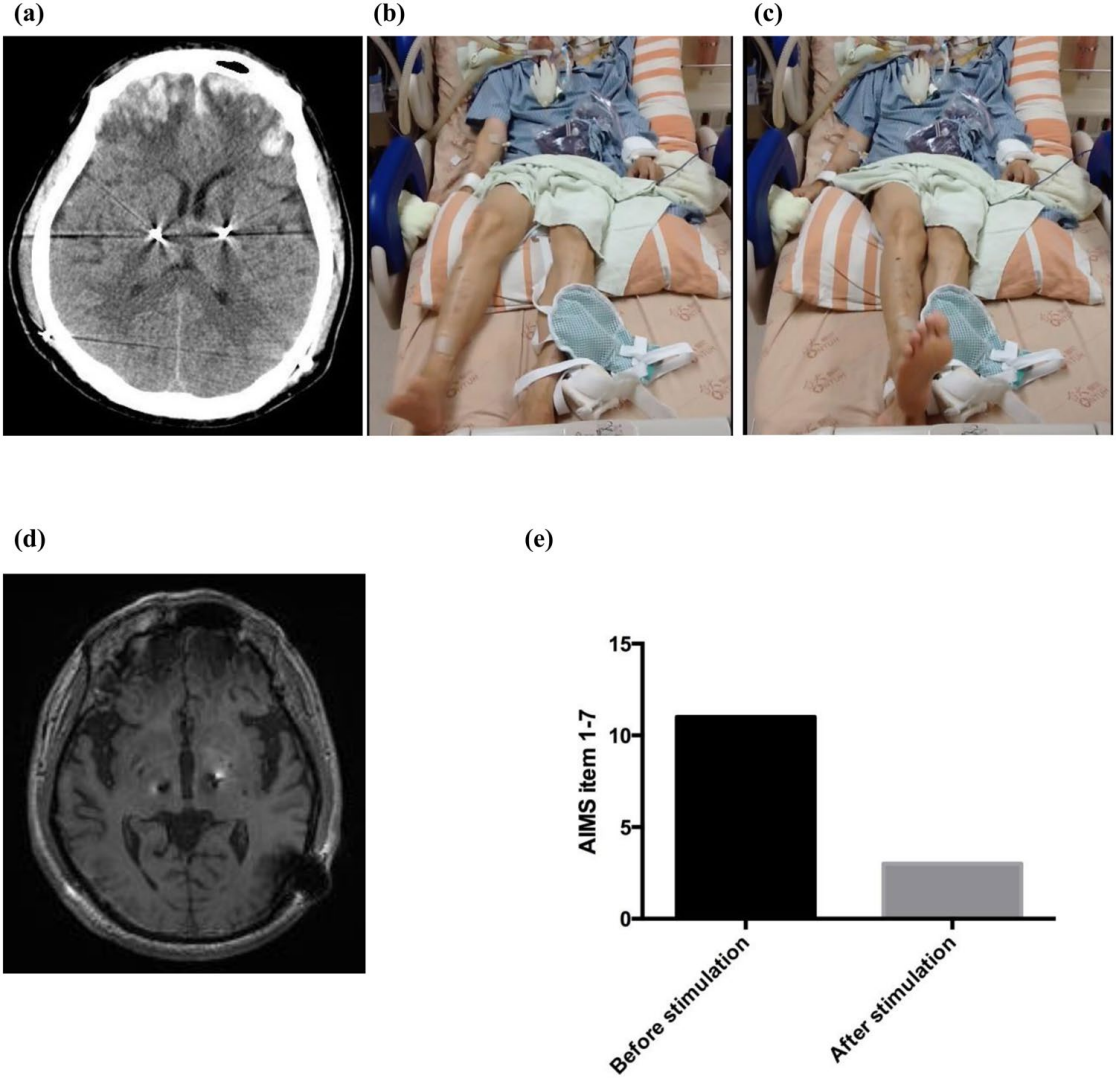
Marked amelioration of hyperkinetic movement in a patient with traumatic intracranial hemorrhage in the bilateral frontal regions by injection of positive current into the STN. (a) CT scan shows bilateral frontal ICH after an accidental fall with severe head injury in the PD patient just implanted with bilateral STN electrodes. (b) and (c) Photos of hemiballistic movement of the right upper and lower limbs of the patient. (d) Location of the DBS electrodes. (e) AIM scores of the patient before, during and after DBS with constant positive current (left STN stimulation with the following settings: 0(−) 1(+)2(+)3(+) 7.5 volts, 450 µs, 130 Hz). The hyperkinetic movements were always markedly inhibited “immediately” upon switching on the DBS but would recur within a few seconds after turning off the DBS. Please refer to the video segment in the supplemental material.
